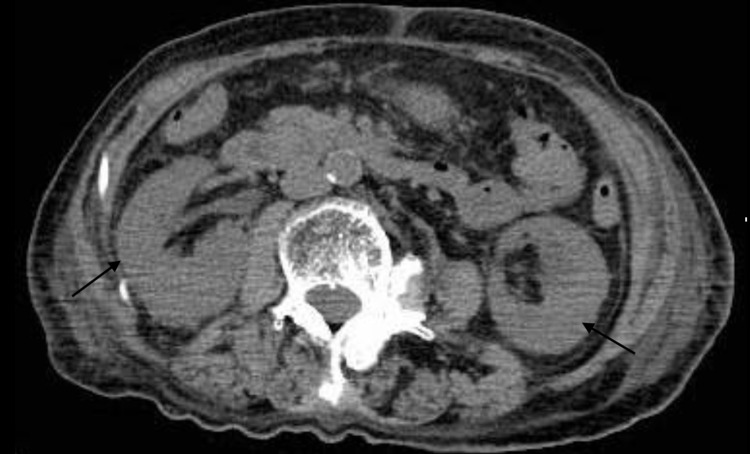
Abdominal CT There is no obvious renal atrophy.
